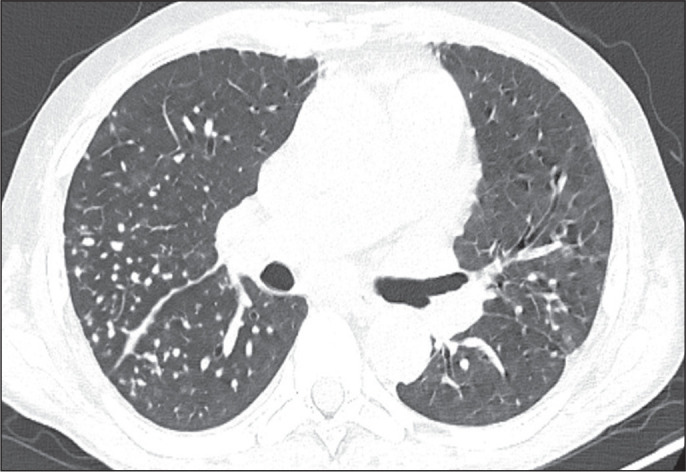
Atypical CT findings. Axial CT scan showing nodules with a centrilobular
